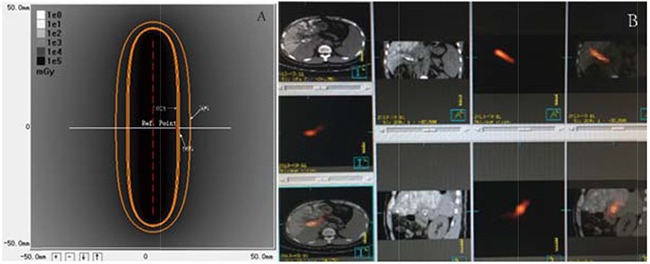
Images of dosimetry of a Iodine-125 seeds strand and SPECT/CT A. Dosimetry of a seed strand containing 16 Iodine-125 seeds. The isodose contours are: 100% (62.5Gy, reference point, red dot), 90% (56.2Gy), and 50% (31.2Gy). The 240 day accumulation dose was141.6Gy. B. Image of a SPECT/CT scan performed 1 day after the procedure. Stent and I-125 seed strands were implanted correctly in the MPV without displacement. Radiation emitted by a I-125 seed strand was distributed homogeneously and completely covered the target lesion.
